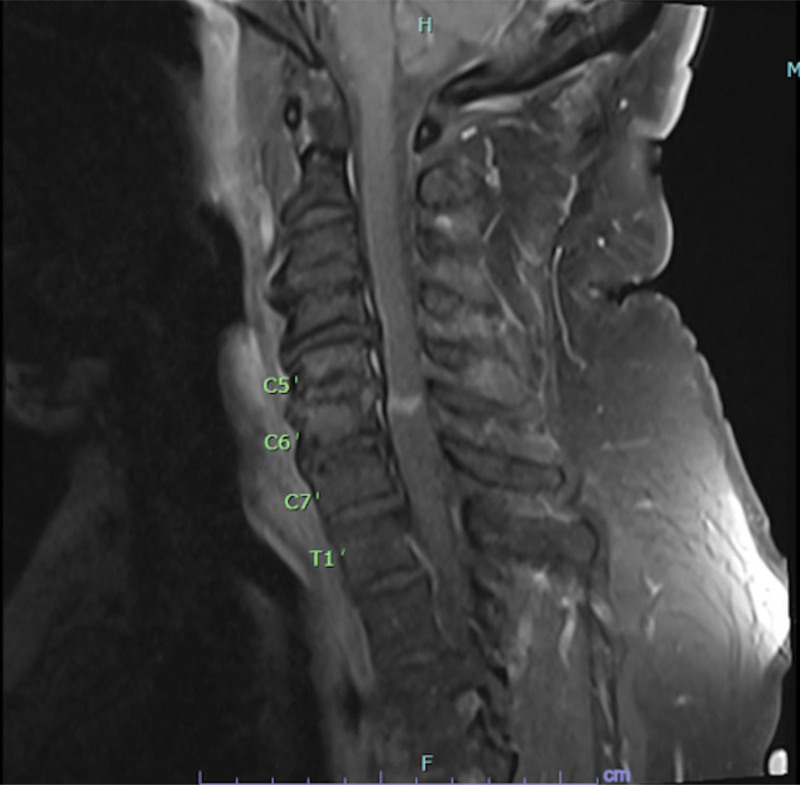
Magnetic resonance imaging of cervical spine with and without contrast showing acute demyelination consistent with transverse myelitis.
